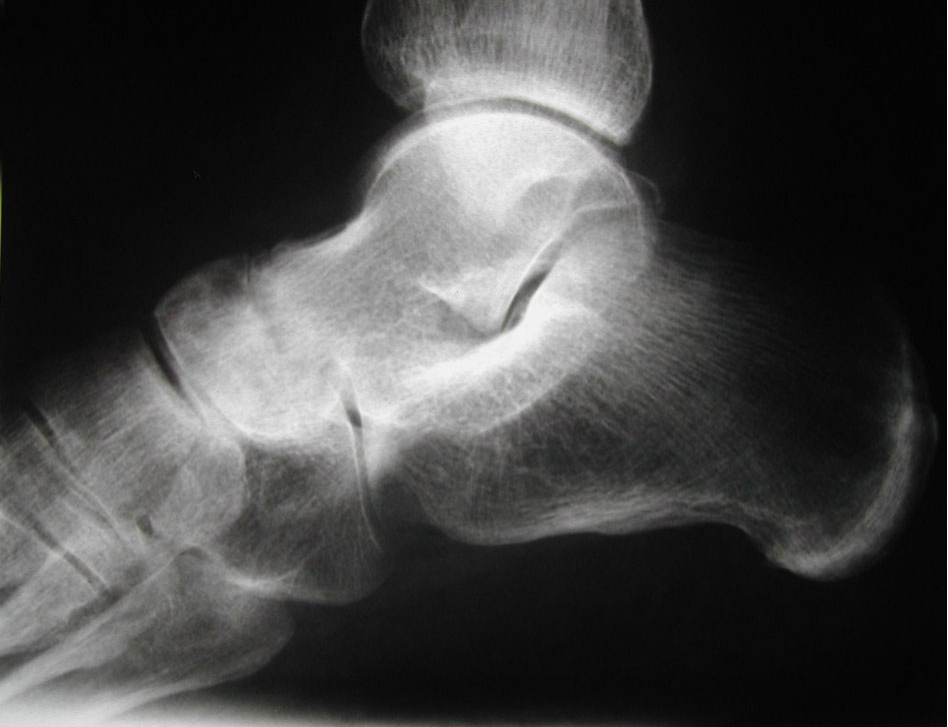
Lateral X-ray of a foot. Lateral X-ray of the foot shows destruction of the talonavicular joint with narrowing of the joint space.
